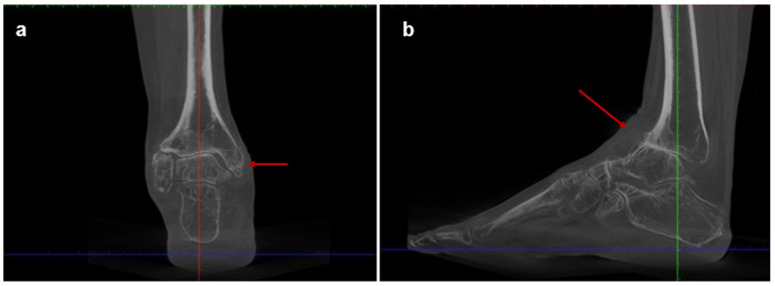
Preoperative weight-bearing computed tomography (WBCT) scans of Case one: (a) coronal and (b) sagittal WBCT views demonstrating advanced tibiotalar osteoarthritis and distal tibial bone irregularities. The arrow in panel (a) indicates advanced tibiotalar osteoarthritis, while the arrow in panel (b) indicates anterior talar shift.
